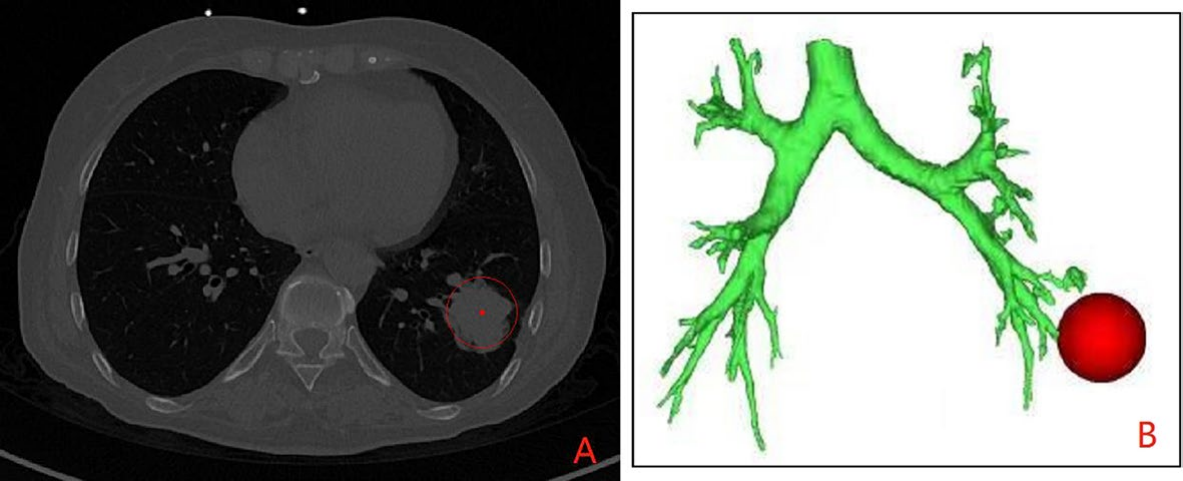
Preoperative location of the tumor (A is the max image of tumor on chest-enhanced CT, B is the three-dimensional reconstruction of bronchi and tumor based on chest-enhanced CT)
